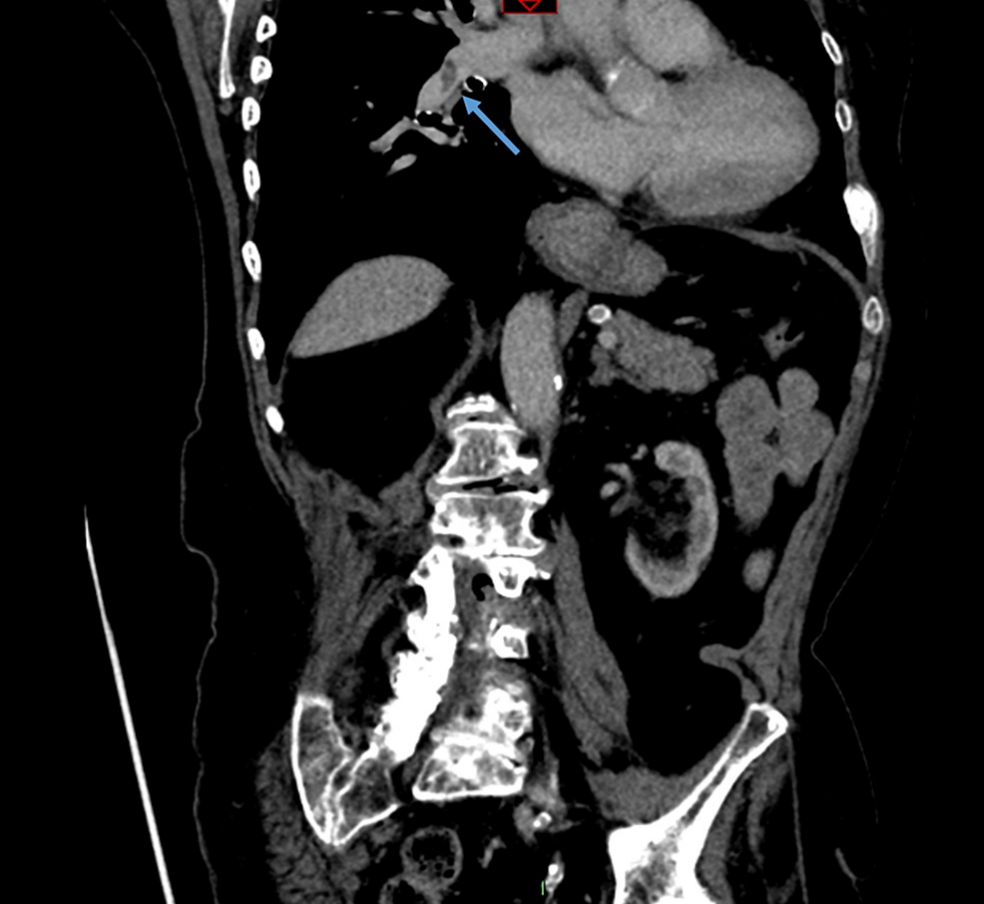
CT scan showing small pulmonary embolus (arrow)
